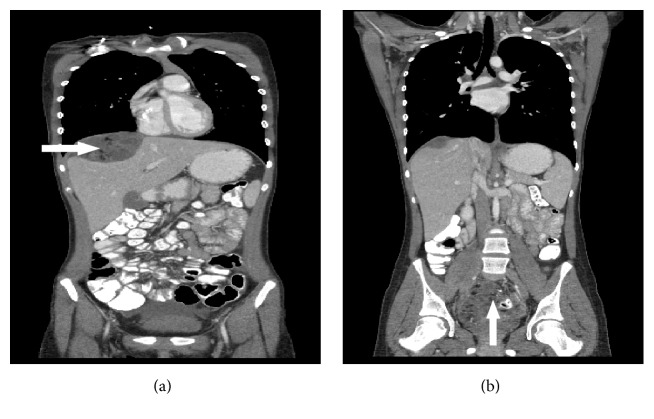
Computed tomography abdomen and pelvis follow-up imaging at 8 months. (a) Large mass occupying the right upper abdominal cavity, revealing multiple new masses containing cystic and necrotic elements surrounding the liver (arrow). Because of tumor growth, the giant mass had compressed the liver parenchyma. (b) Pelvic tumor is demonstrated as well (arrow).
